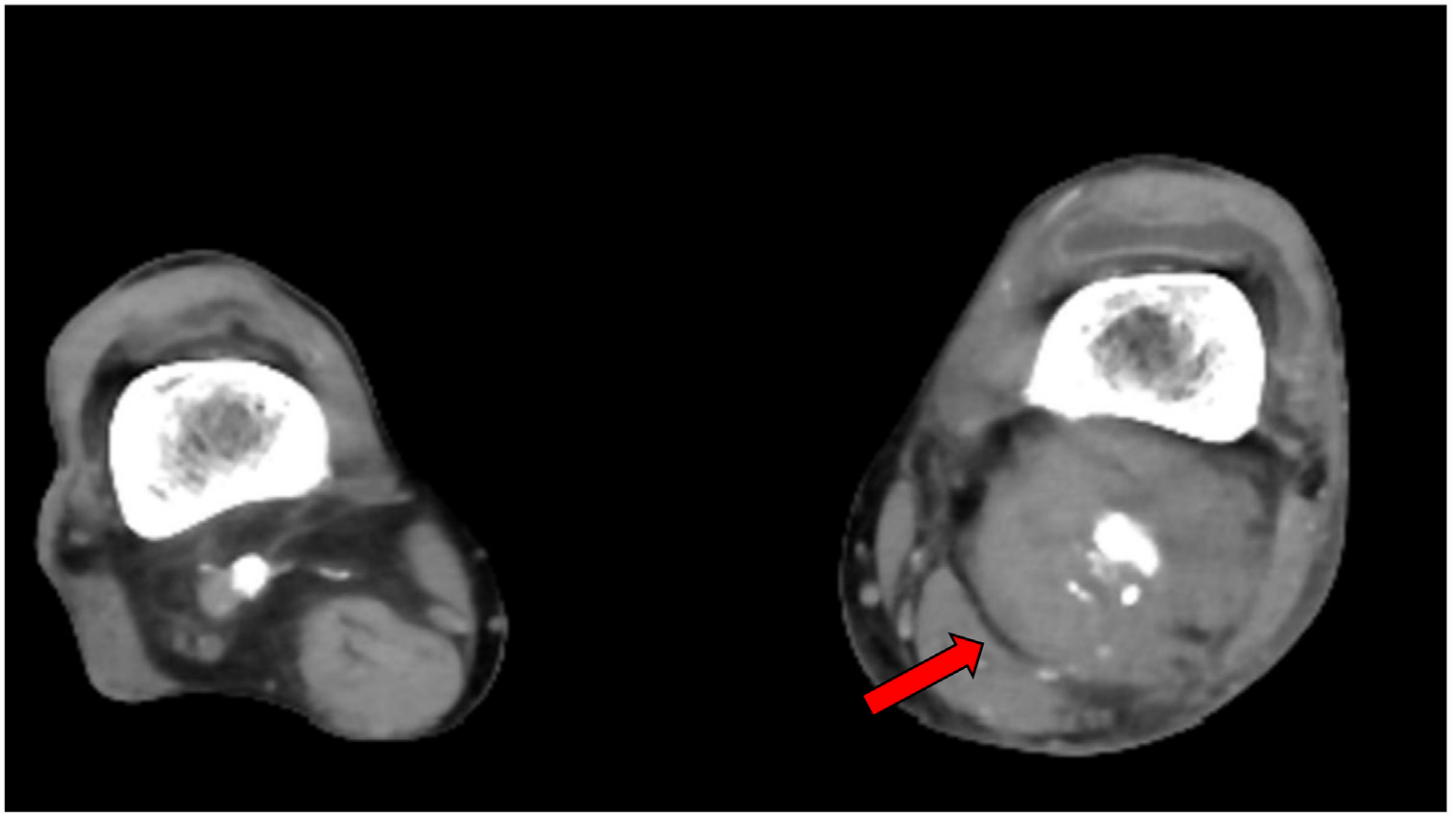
Popliteal artery imaged by contrast-enhanced computed tomography. Contrast-enhanced computed tomography reveals formation of a pseudoaneurysm with a maximum dimension of 54 × 55 mm in the left popliteal artery (red arrow).
